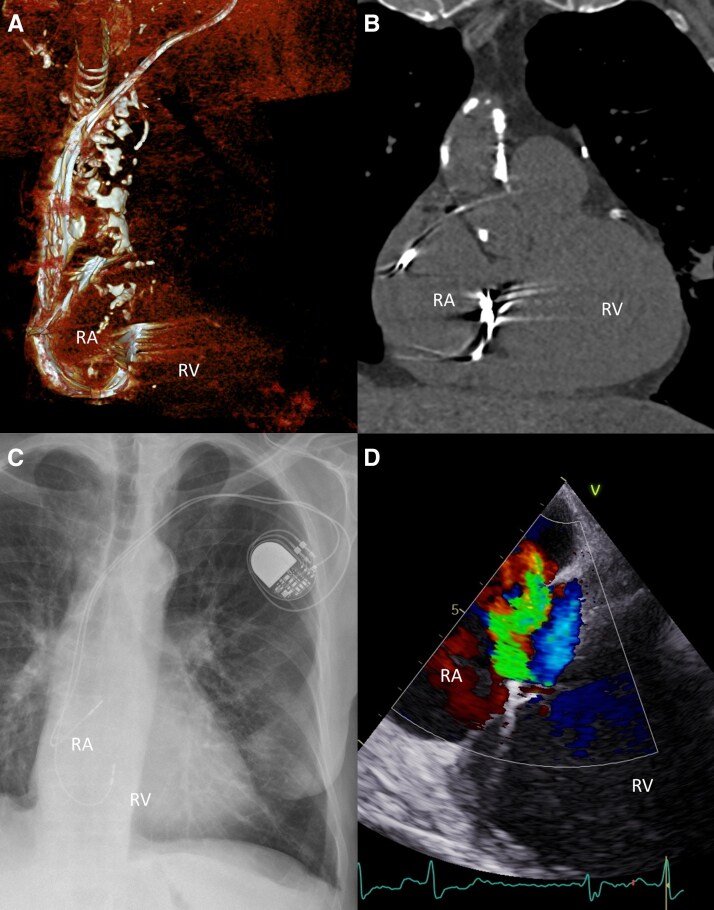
Patient 3. Malpositioned ventricular lead, looped and hypermobile at the tricuspid annulus. *(A)* 3D CT reconstruction. *(B)* CT coronal plane. *(C)* Chest X-ray. *(D)* Transthoracic echocardiography (TTE) showing eccentric regurgitant jet on colour Doppler. RA—right atrium; RV—right ventricle.
